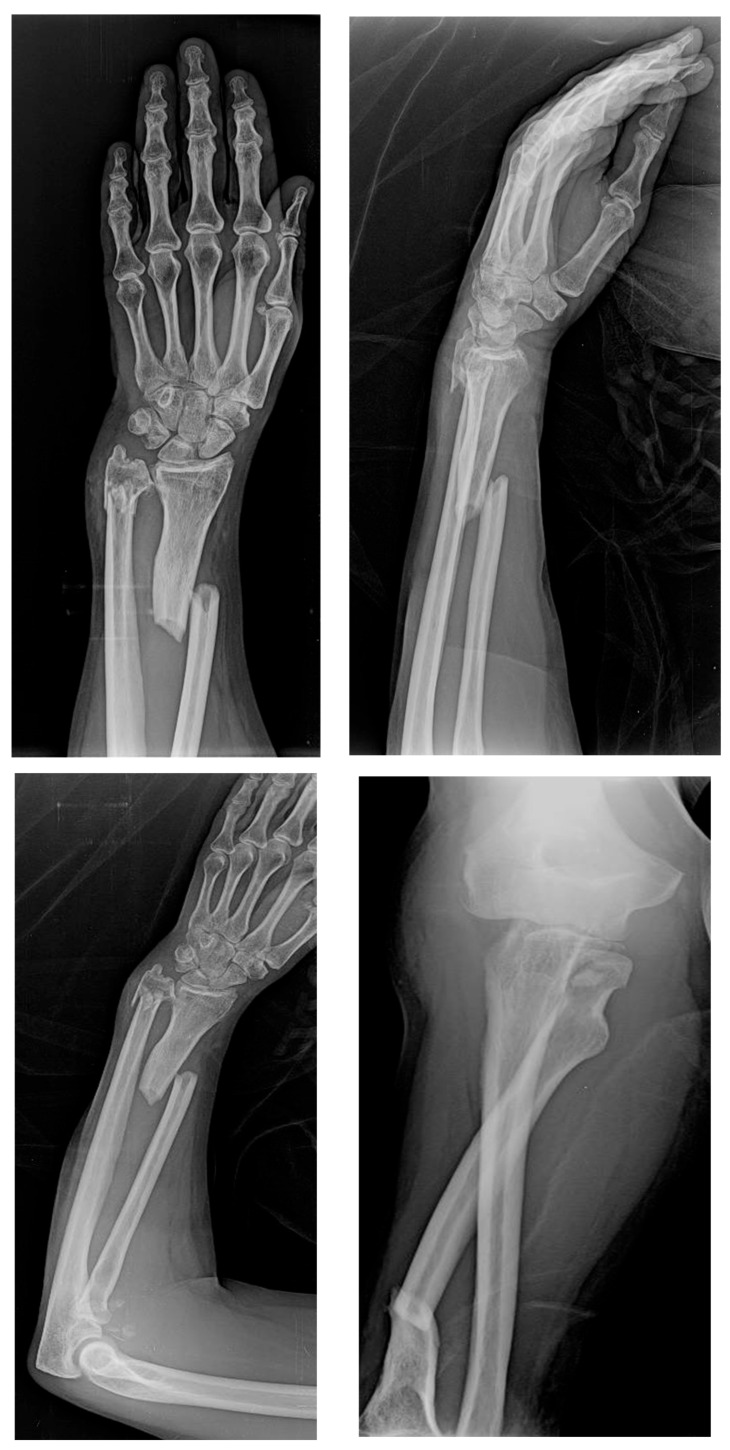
X-rays performed at the emergency room entrance.
